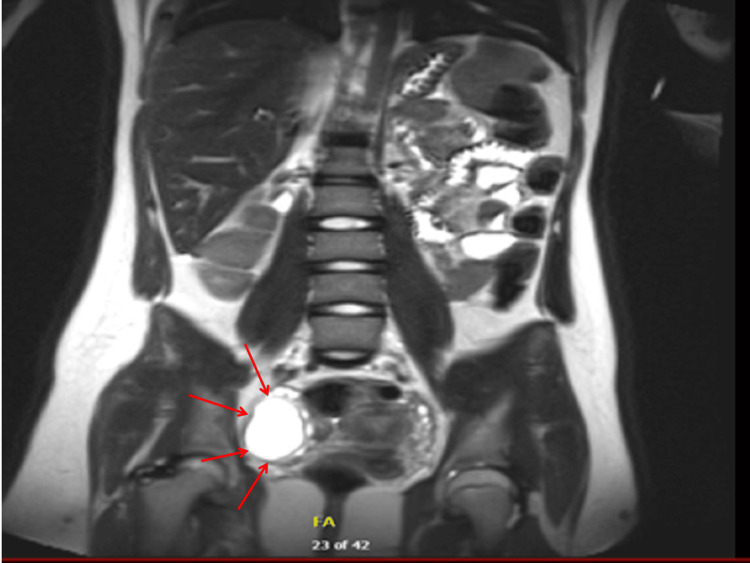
Right ovarian tumor MRI coronal image (Case 1) Coronal T2-weighted MRI showing a well-defined, approximately 3-cm right ovarian cystic lesion. The lesion exhibits both fluid and soft-tissue components consistent with a mature cystic teratoma (dermoid cyst), located lateral to the uterus and adjacent to the pelvic brim.
